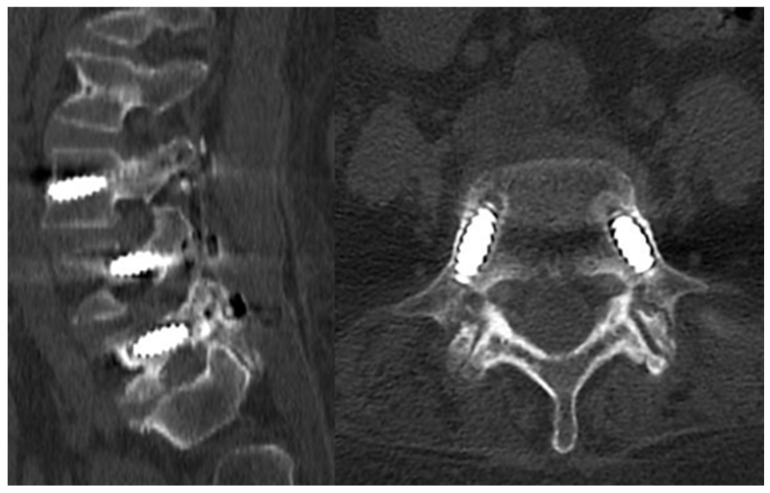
Sagittal and axial section CT image of a patient who developed pseudoarthrosis during postoperative follow-up.
